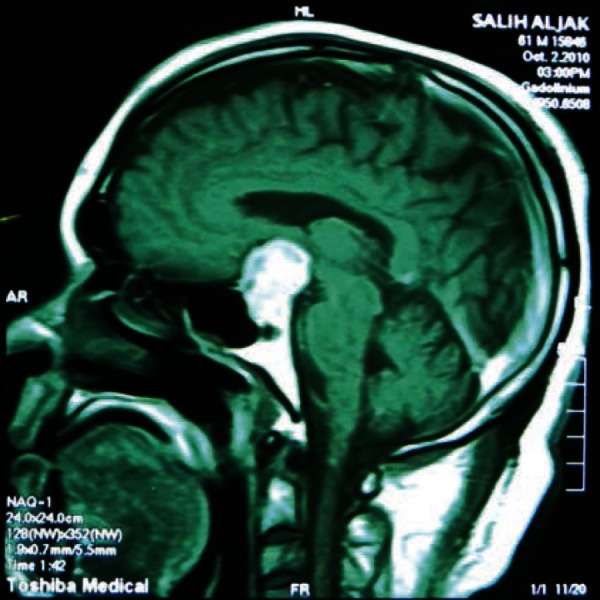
An MRI revealed a large pituitary adenoma responsible for visual loss in both eyes (on top of his POAG).
